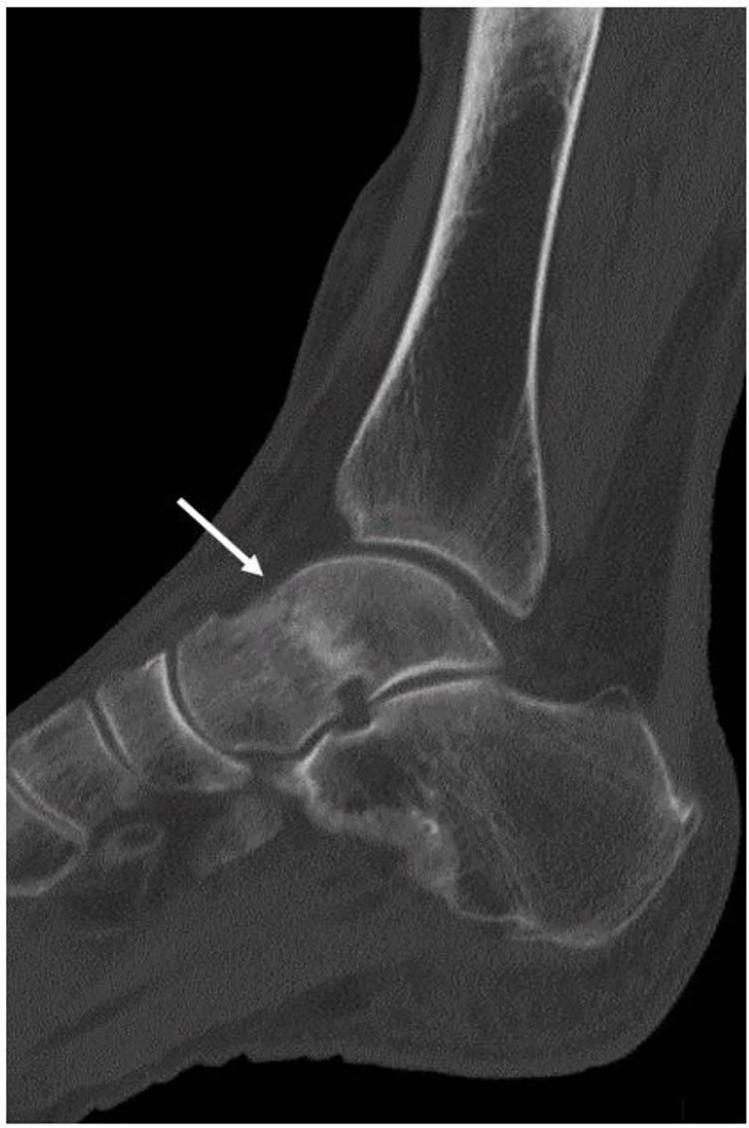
Although a non-contrast CT scan of the sagittal view demonstrated a fracture line in the talar neck (arrow), the bone fragment was not dislocated.
